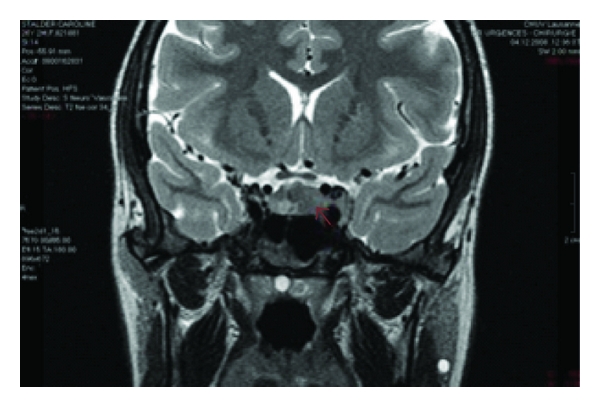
Cranial computed tomography shows a mass of 20 × 20 × 13 mm in the left part of the sella turcica.
